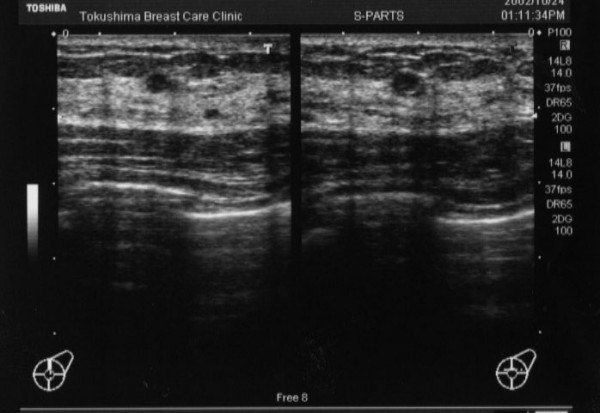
Ultrasonography showed a hypo-echoic legion of 3 mm in diameter in C region of the left breast.
